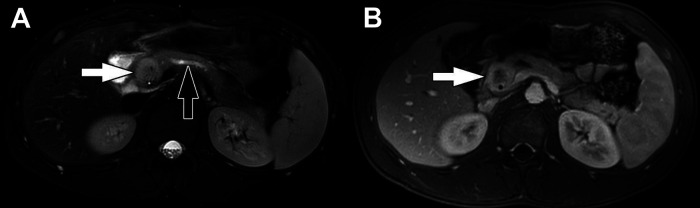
Inflammatory myofibroblastic tumor. (A) Axial T2-weighted fat-saturated MRI and (B) Axial T1-weighted fat-saturated post-contrast MRI show a mass in the head of the pancreas (white arrows). The mass is heterogeneously T2-weighted hyperintense with heterogenous enhancement following contrast administration. Lack of central enhancement suggests necrosis. There is associated dilation of the upstream pancreatic duct (black arrow) due to obstruction by the mass.
